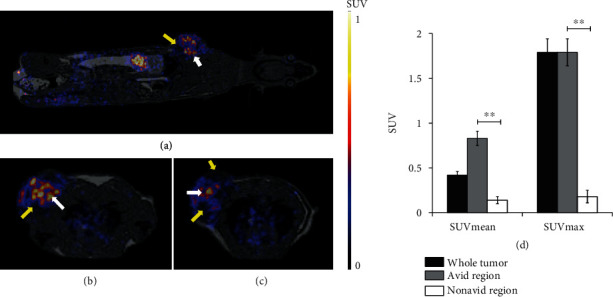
In vivo* PET/MRI imaging of CD13 expression in subcutaneously transplanted He/De tumors. Representative coronal (a) and axial (b, c) decay-corrected PET/MRI images of subcutaneously transplanted He/De tumors 13 days after tumor induction and 90 min after intravenous injection of ^68^Ga-NOTA-c(NGR). White arrows: CD13 positive, avid regions; yellow arrows: nonavid regions. (d) Quantitative image analysis of heterogenous He/De tumors (*n* = 15). Significance level: *p* ≤ 0.01 (∗∗). Data is presented as the mean ± SD.
